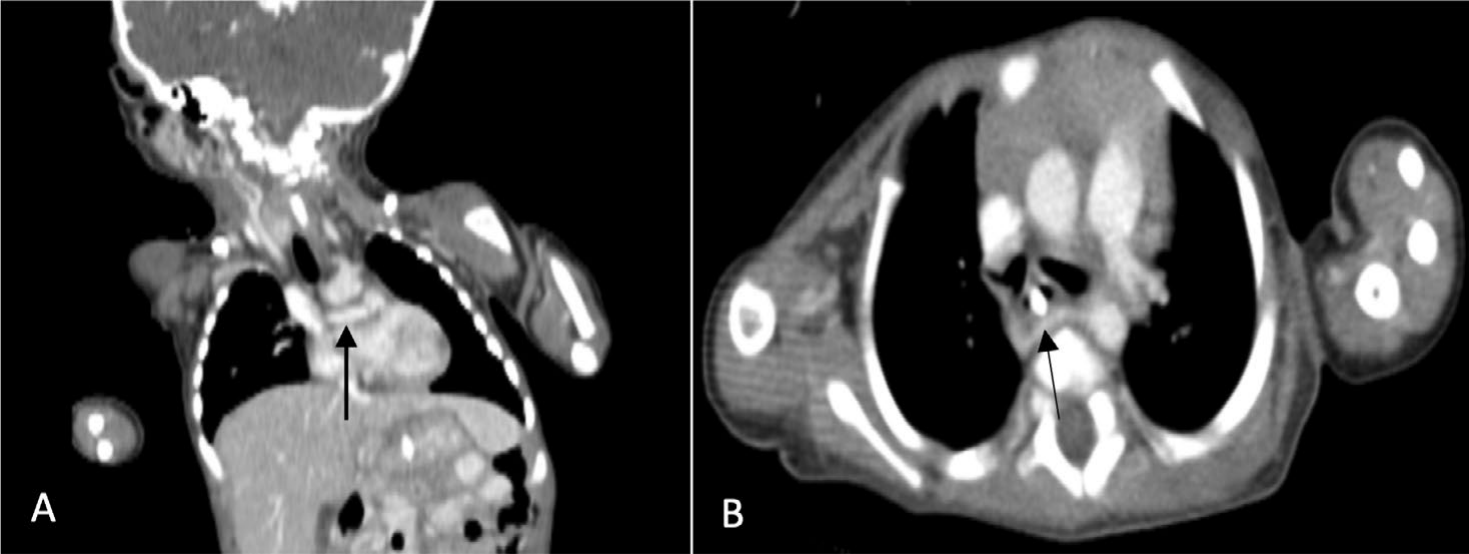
Neck and chest CT scan: white arrow indicates the retroesophageal course of the aberrant right subclavian artery (A, coronal; B, axial).
